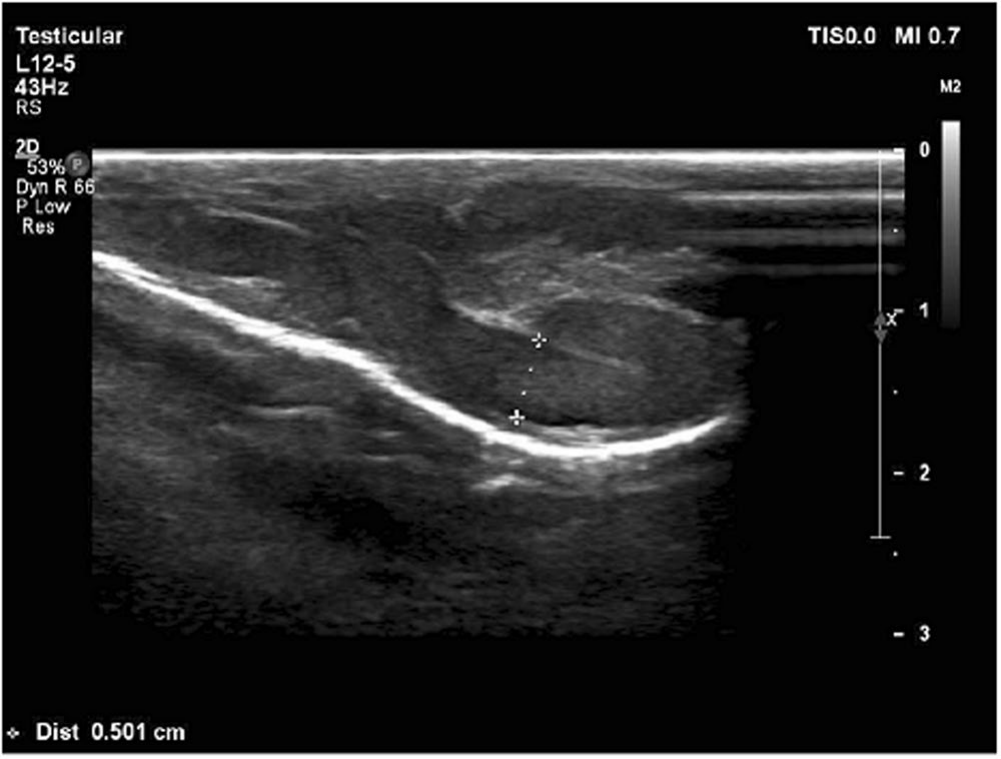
Stasis pattern: sluggish flow in gray-scale ultrasound with no color during rest or Valsalva maneuver.
